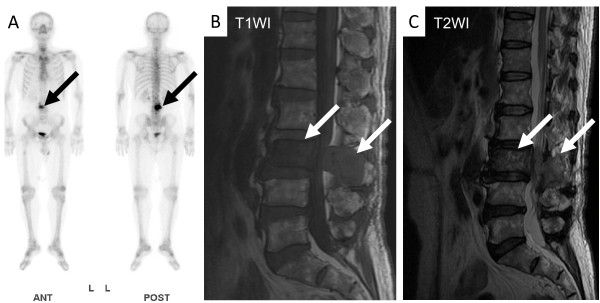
Bone scintigraphy (A) and magnetic resonance imaging (B, C) showed osteoblastic lumbar spine metastases (arrows).
